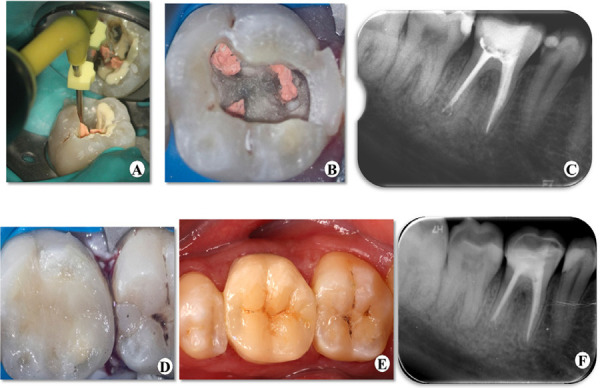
(A) A heated plugger-Alphall used to remove coronal portion of gutta-percha. (B) Pulp chamber floor showing the canal orifice after the obturation.(C) Post obturation radiograph showing the completely filled canal. (D) Postoperative clinical view of tooth #30 after restoration. (E) Occlusal view of the occlusal surface of tooth #30 with onlay. (F) Three-year-follow up radiograph showing no radiographic changes.
